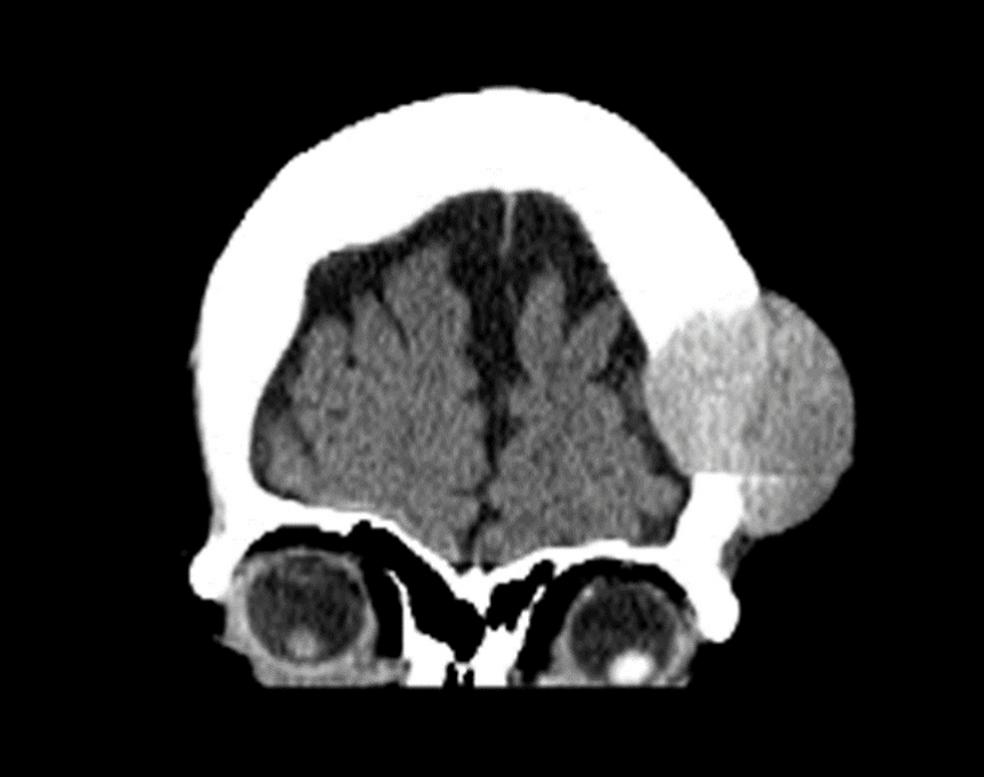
CT head of mass (coronal view)
